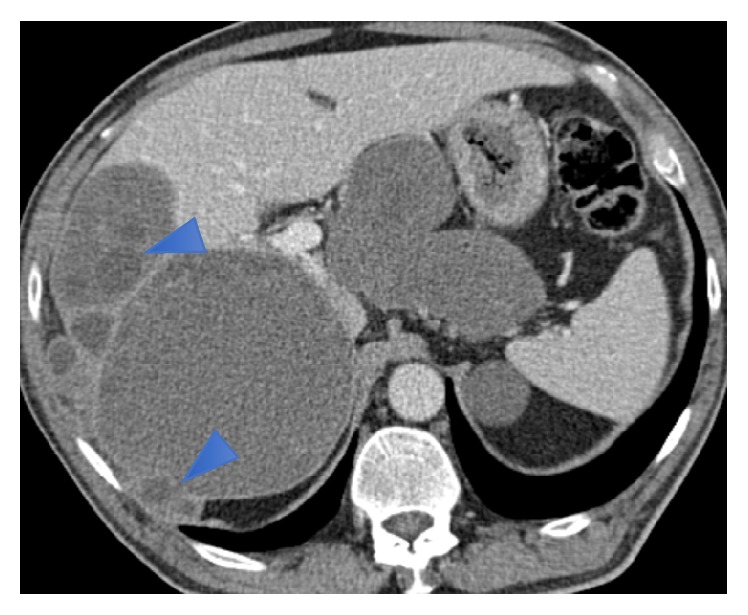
Axial contrast enhanced CT image nicely shows the hypodense lesions with water density and multiple smaller cysts at the periphery of the larger cysts (daughter cysts, arrowheads).
